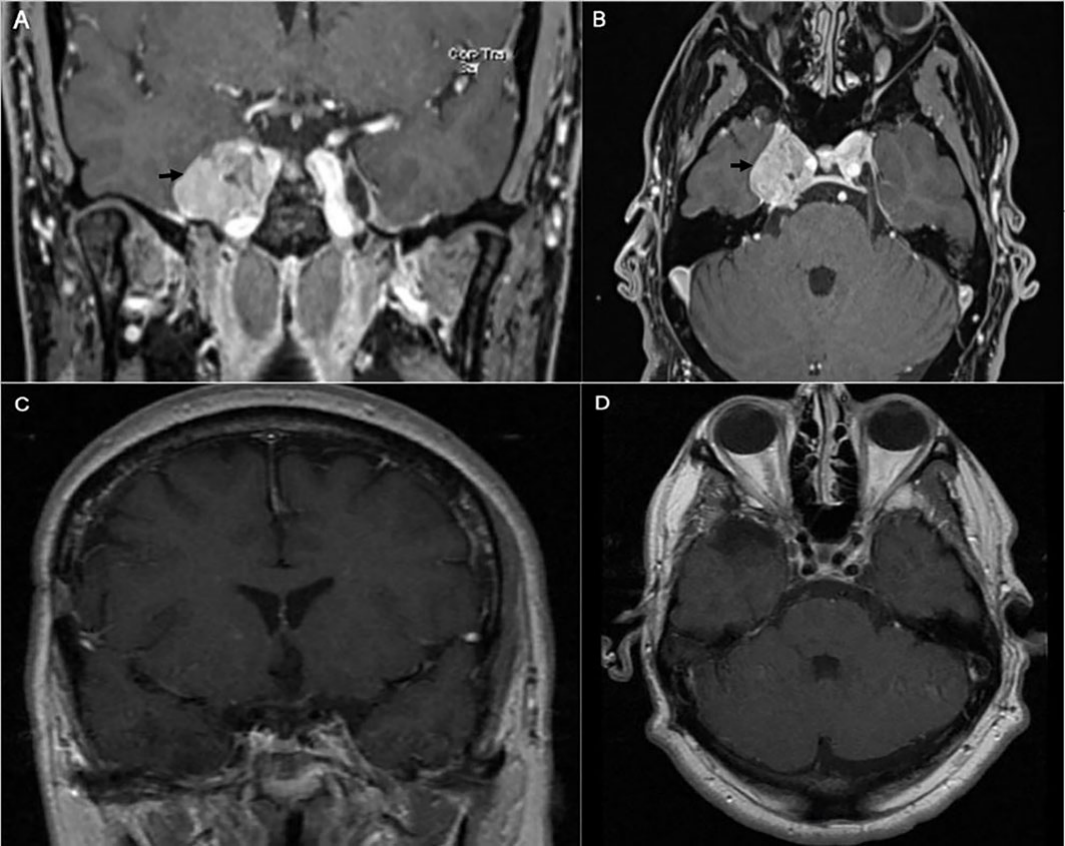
Preoperative coronal (A) and axial (B) brain MRI with contrast showing heterogeneous enhancing tumor involving the right cavernous sinus (arrow). The patient is known to have right cavernous sinus meningioma for which he had stereotactic radiosurgery 4 years prior to presentation. Postoperative coronal (C) and axial (D) brain MRI with contrast showing gross total excision of the meningioma.
